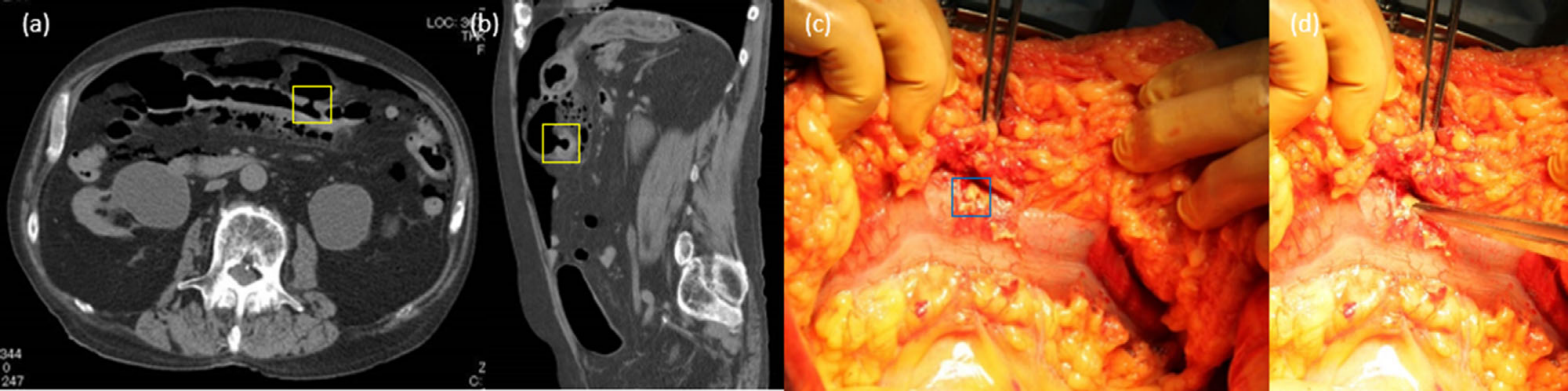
Computed tomography showed free air at the transverse colon perforation site. (a) Axial image. (b) Sagittal image. (c) Intraoperative photograph. The blue rectangle indicates the perforation site. (d) Tweezers are pointed at the perforation site.
